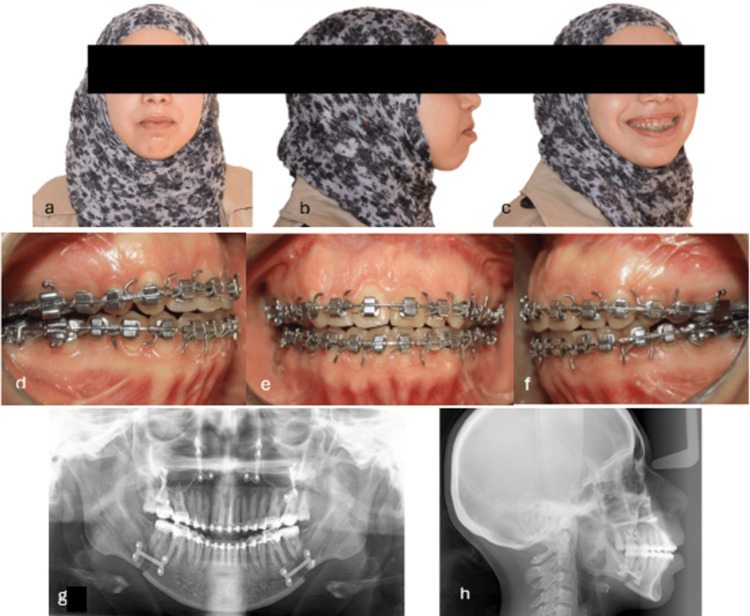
Post-surgical records at two-month follow-up. (a) Frontal extraoral photograph. (b) Right profile extraoral photograph. (c) Smiling extraoral photograph. (d) Right buccal intraoral view. (e) Frontal intraoral view. (f) Left buccal intraoral view. (g) Panoramic radiograph. (h) Lateral cephalometric radiograph.
